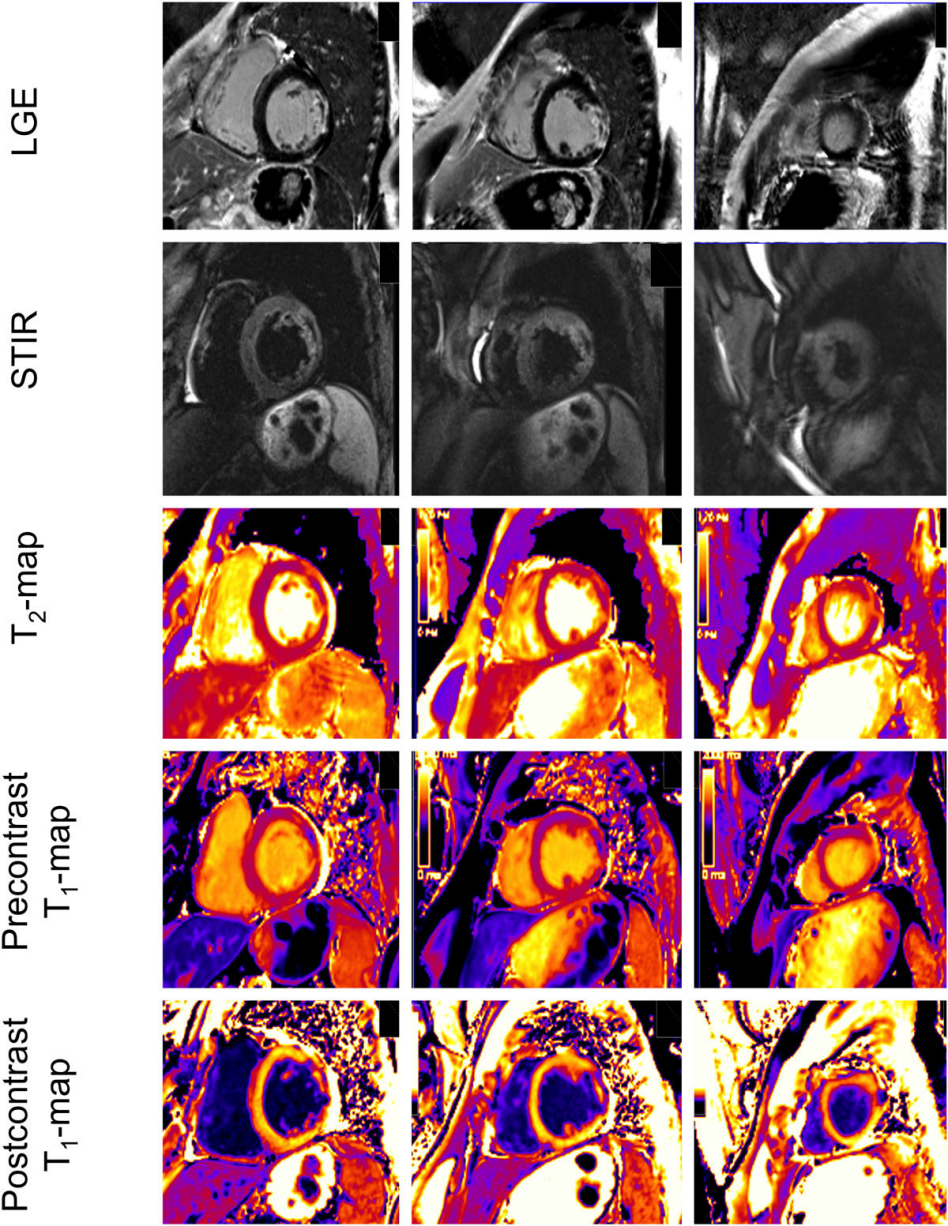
# Differentiation of acute and chronic myocardial infarction using T2-weighted imaging, late enhancement and T1 and T2 mapping - a pilot study at 3T
